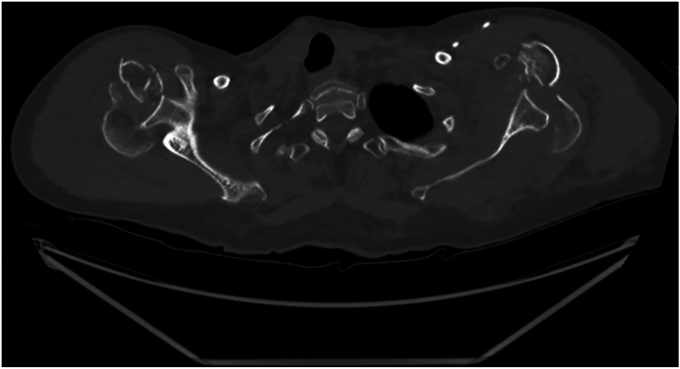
CT-scan (axial view) of bilateral shoulders performed in Emergency Department, showing bilateral posterior fracture-dislocation. *CT*, computed tomography.
